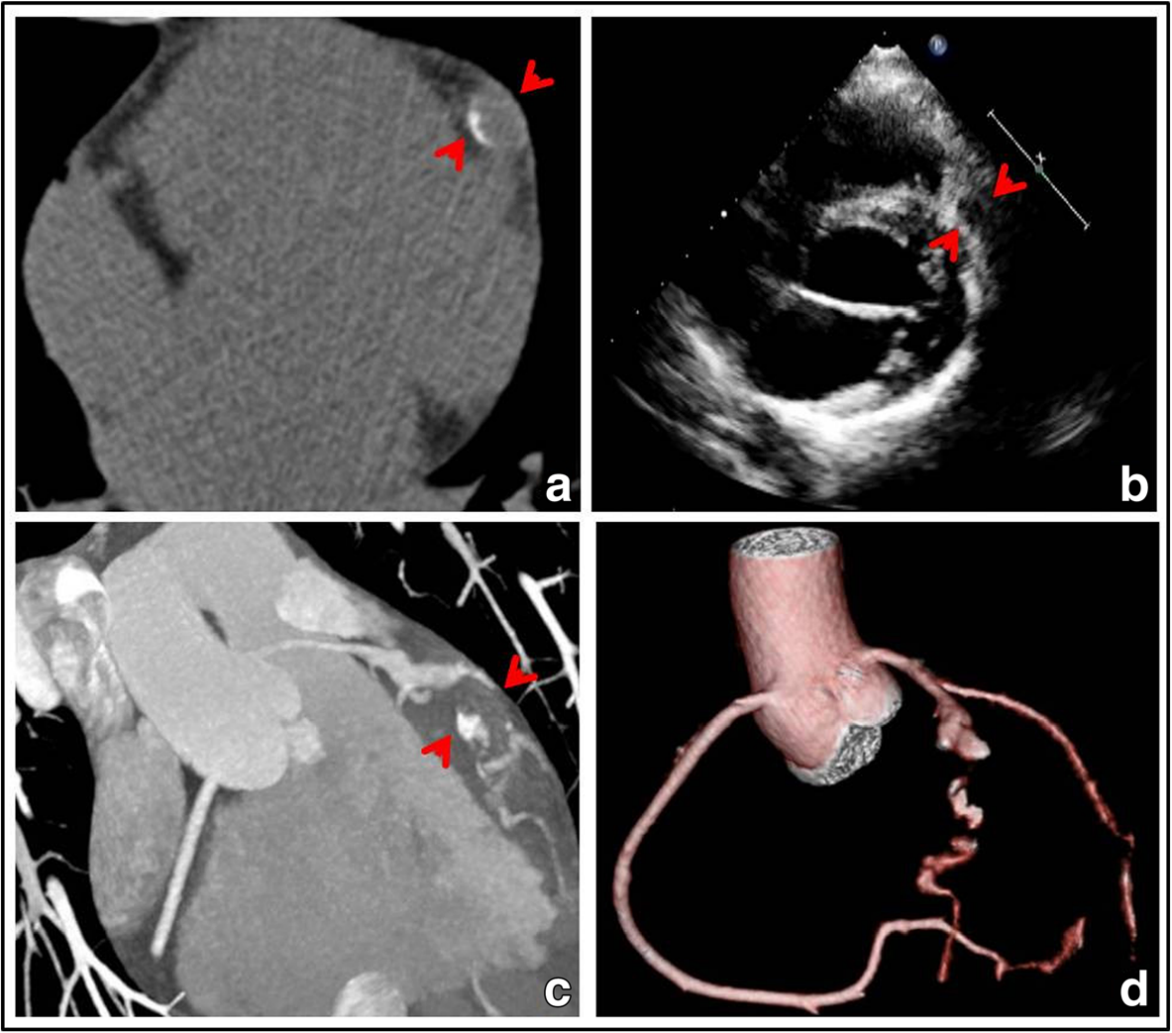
Coronary artery aneurysm of the left anterior descending artery. a Chest computer tomography (CT): ringed calcification with 9.8 mm diameter in the left anterior descending (LAD) artery. b Trans-thoracic echocardiography: the proximal aneurismal dilation of the LAD artery. c Coronary CT angiography(CTA) image in orthogonal plane: LAD artery aneurysm. d Coronary CTA with three-dimensional volume rendering: multiple giant aneurysms with lumen occlusion and distal re-canalization in the LAD artery (arrows)
